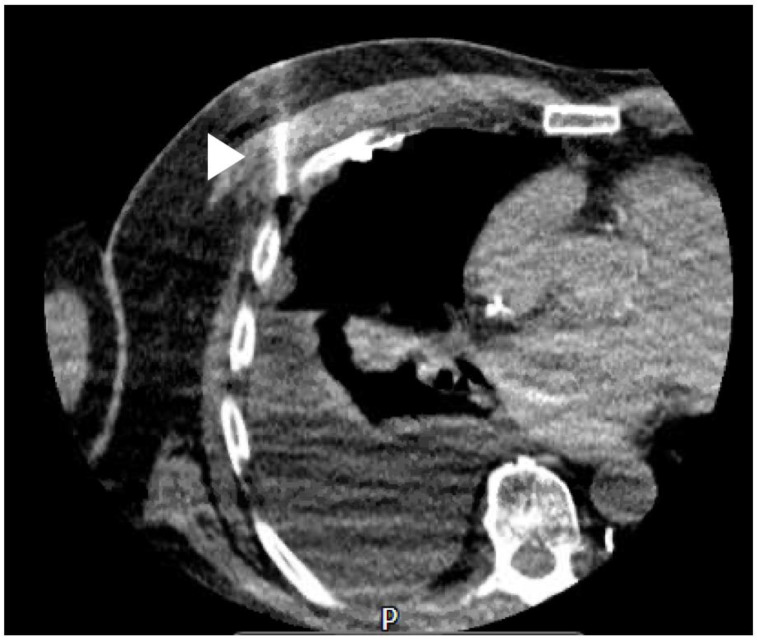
Image of a CT-guided needle biopsy; white arrow = needle.
